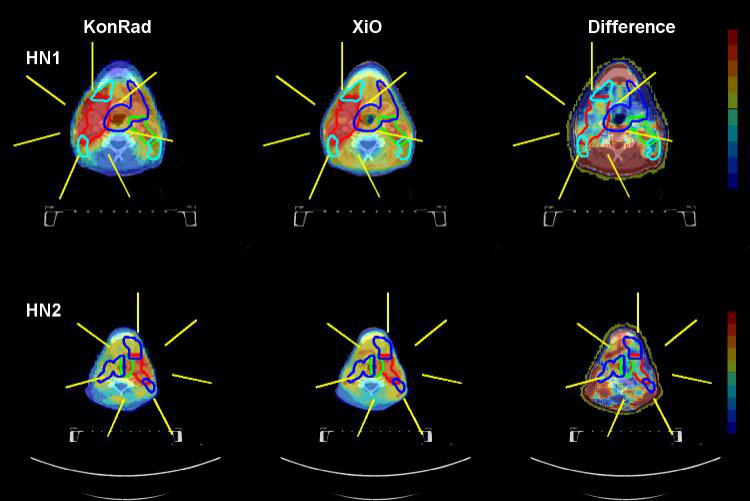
Beam arrangement and dose distribution for cases HN1 (top row) and HN2 (bottom row) from the KonRad (left column) and XiO (middle column) systems. The beam directions are indicated as yellow lines on a transverse CT slice through the isocenter. For HN1 the contours of ${\text{PTV}}_{70}$, ${\text{PTV}}_{65.8}$, ${\text{PTV}}_{63}$, and ${\text{PTV}}_{57.8}$ are shown in red, green, blue, and cyan. For case HN2 the contours of ${\text{PTV}}_{62.4}$, ${\text{PTV}}_{57.6}$, and ${\text{PTV}}_{53.4}$ are shown in red, green, and blue. The dose distribution for the two plans is shown as a color wash overlay. The right column shows dose difference maps. The color wash ranges from +5 Gy in dark red to −5 Gy in dark blue, where positive numbers indicate a higher dose value in the XiO plan.
